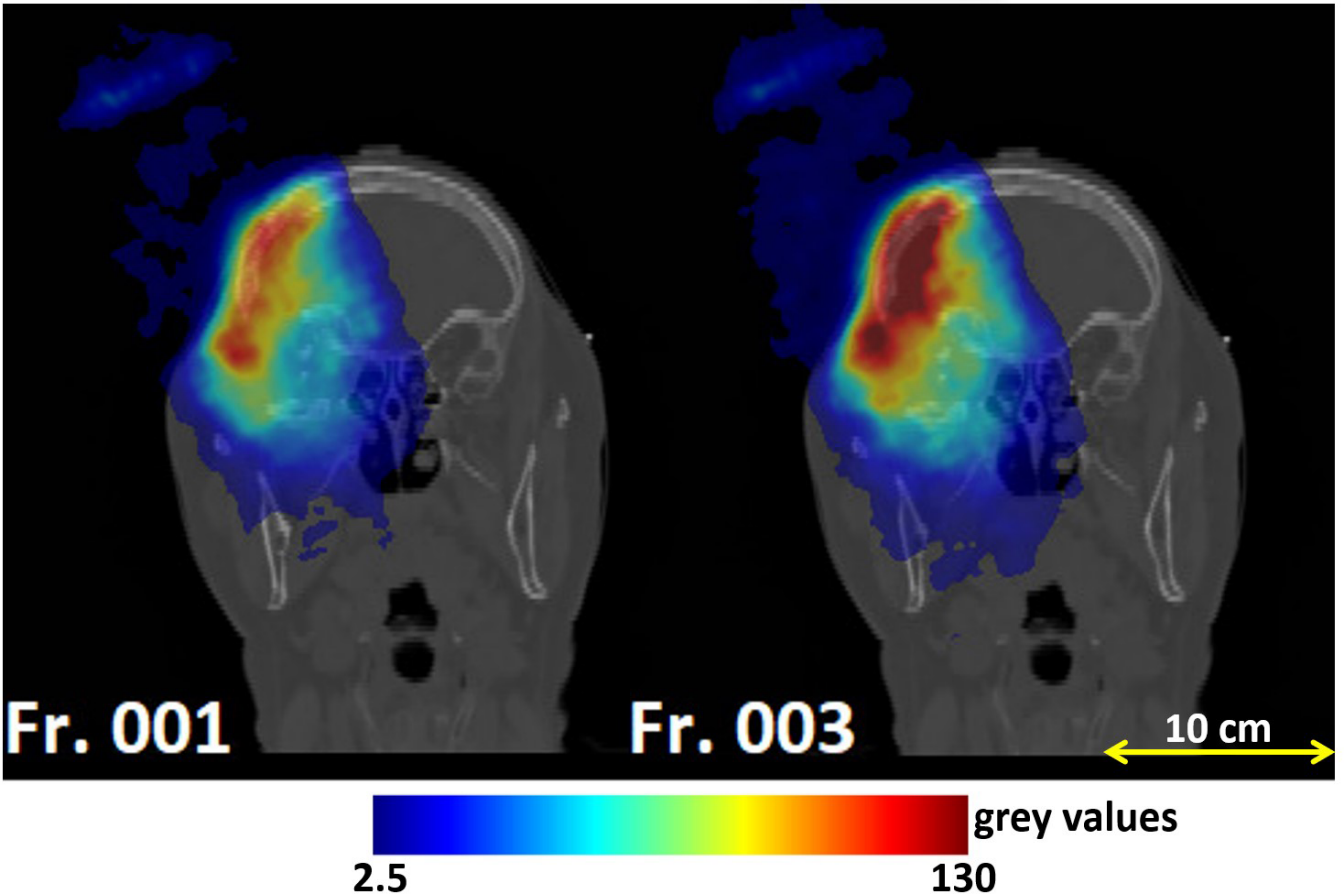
Coronal views of the planning CT of patient 004P: reconstructed PET images corresponding to the monitored fractions 1 and 3. The left and right image had different dose delivery and acquisition times, resulting in different activities. Fraction 3 had to be excluded because of the larger activity compared to the other fractions.
